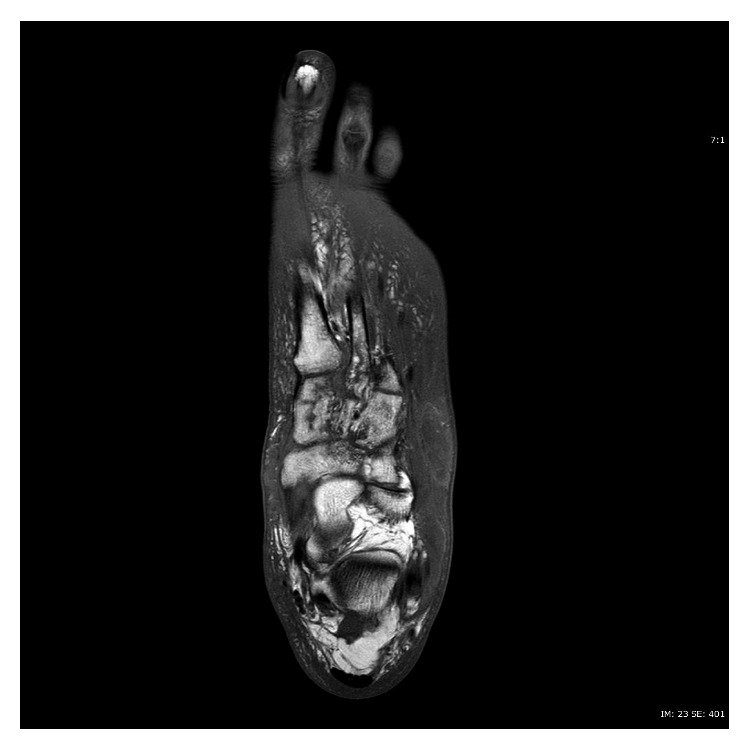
T1-weighted axial image of MRI of left foot without contrast, showing decreased signals (hypointensities) of cuneiforms and metatarsals, reflecting medullary edema and osteomyelitis.
